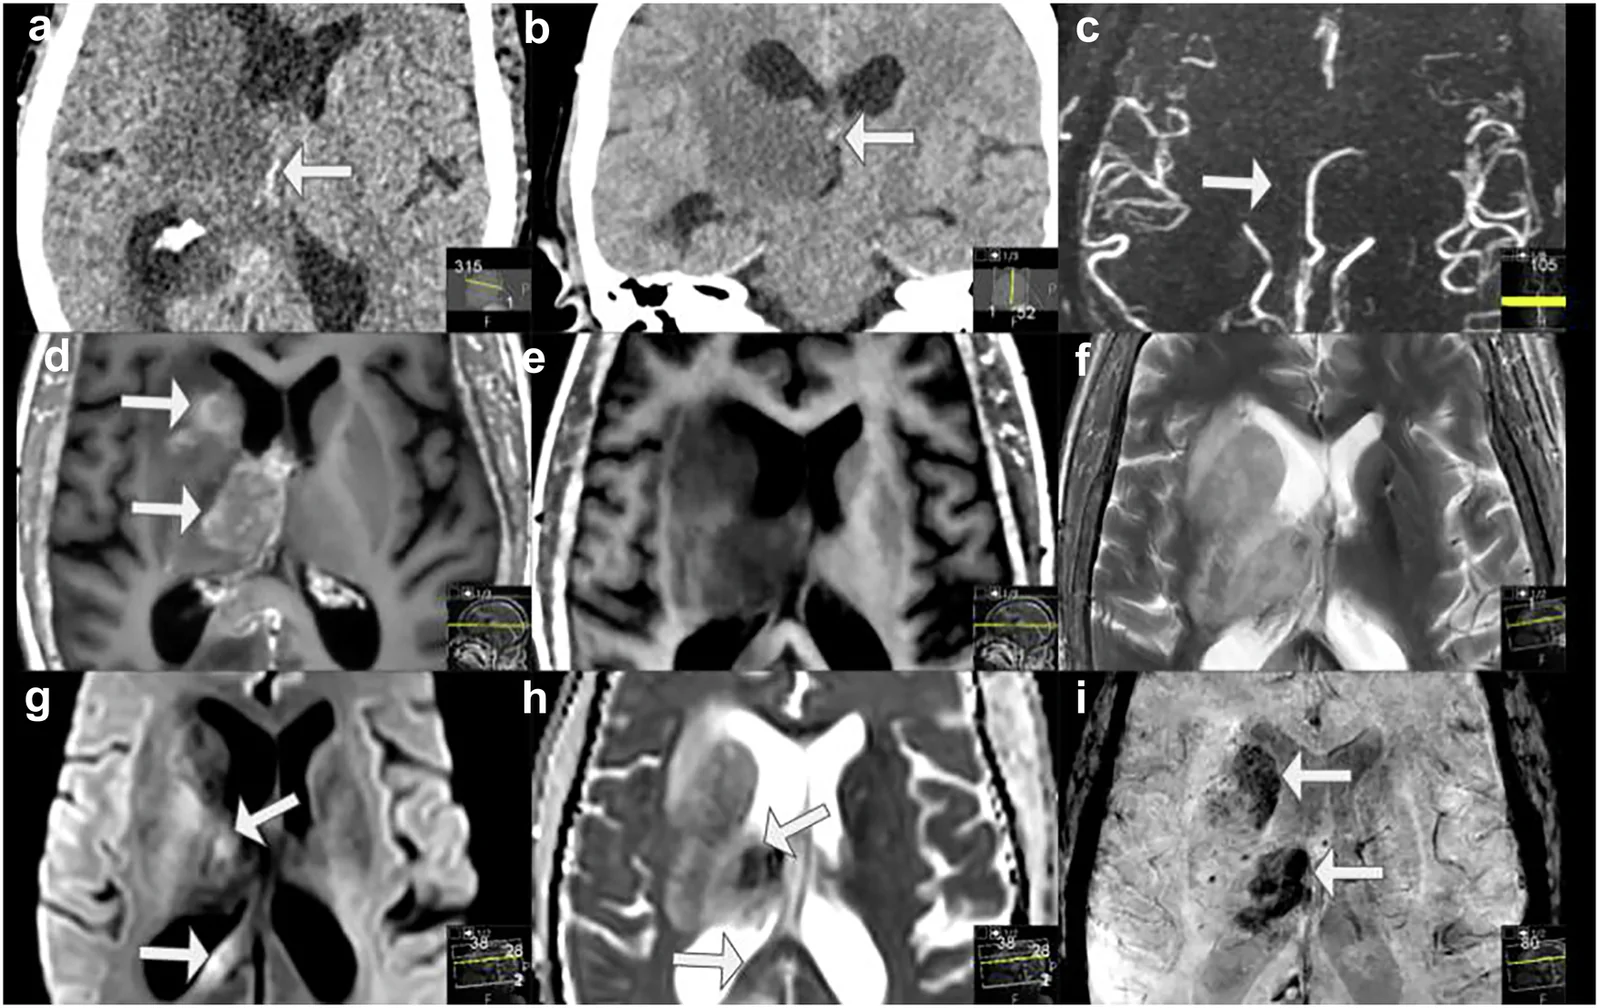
Right internal cerebral vein thrombosis with venous infarction mimicking malignancy. Upper row: a Non-enhanced axial CT and (b) coronal CT show hyperattenuating right internal cerebral vein (arrows) with central hemispheric edema. c CT venography demonstrates absent opacification of the right internal cerebral vein (arrow), confirming thrombosis. Middle row: d Non-enhanced T1 and (e) post-gadolinium T1 show enhancement in deep gray nuclei (arrows), with (f) T2-weighted imaging demonstrating surrounding edema. Lower row: (g) DWI and (h) ADC map show patchy restricted diffusion (arrows) indicating cytotoxic edema, and (i) SWI demonstrates signal loss from hemorrhagic transformation (arrows). This constellation of findings mimicked malignancy (lymphoma or glioma), but biopsy confirmed venous infarction secondary to unilateral deep venous thrombosis
